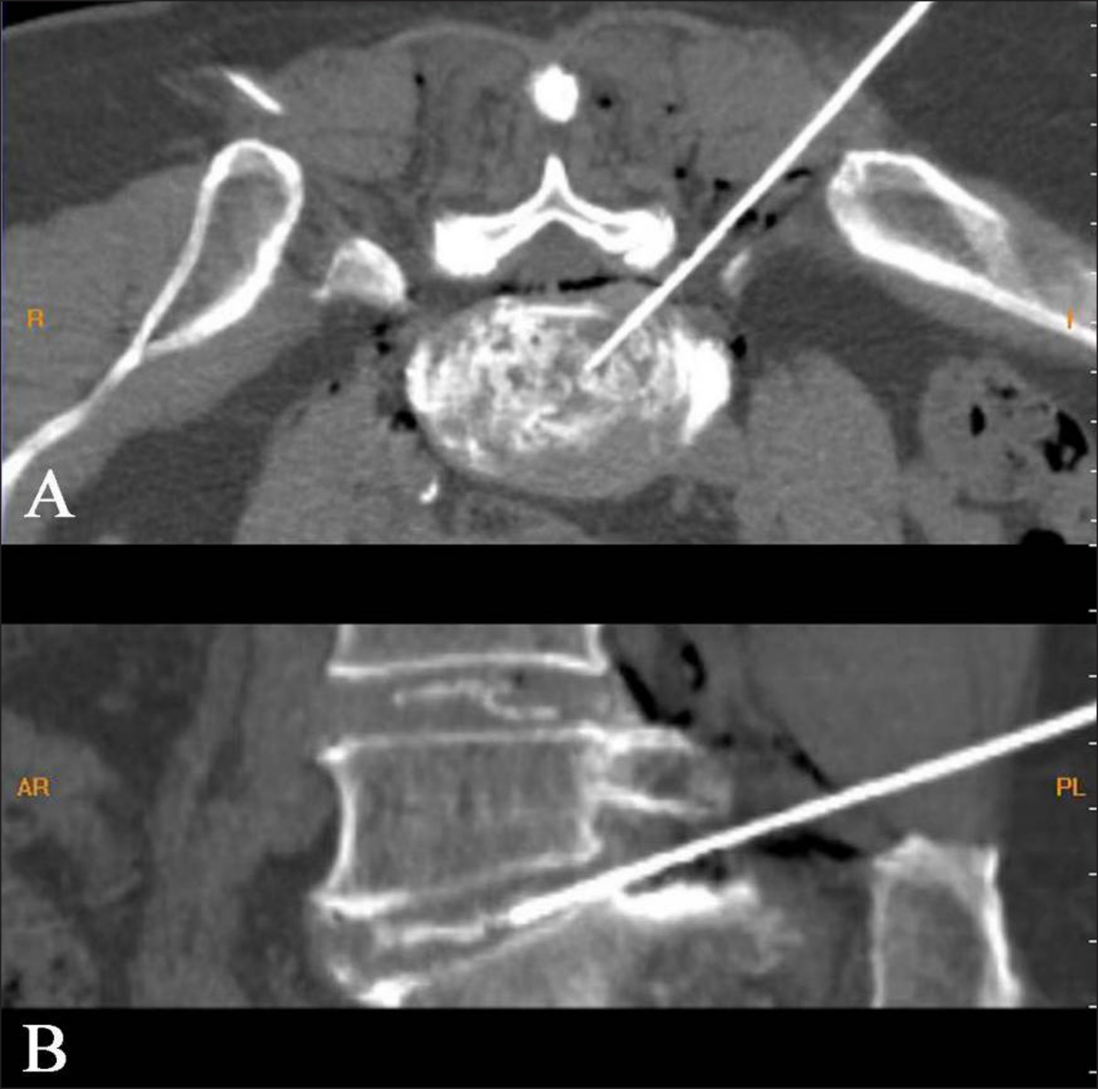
Intraoperative: Axial (A) and sagittal (B) CT images of collagenase treatment.
